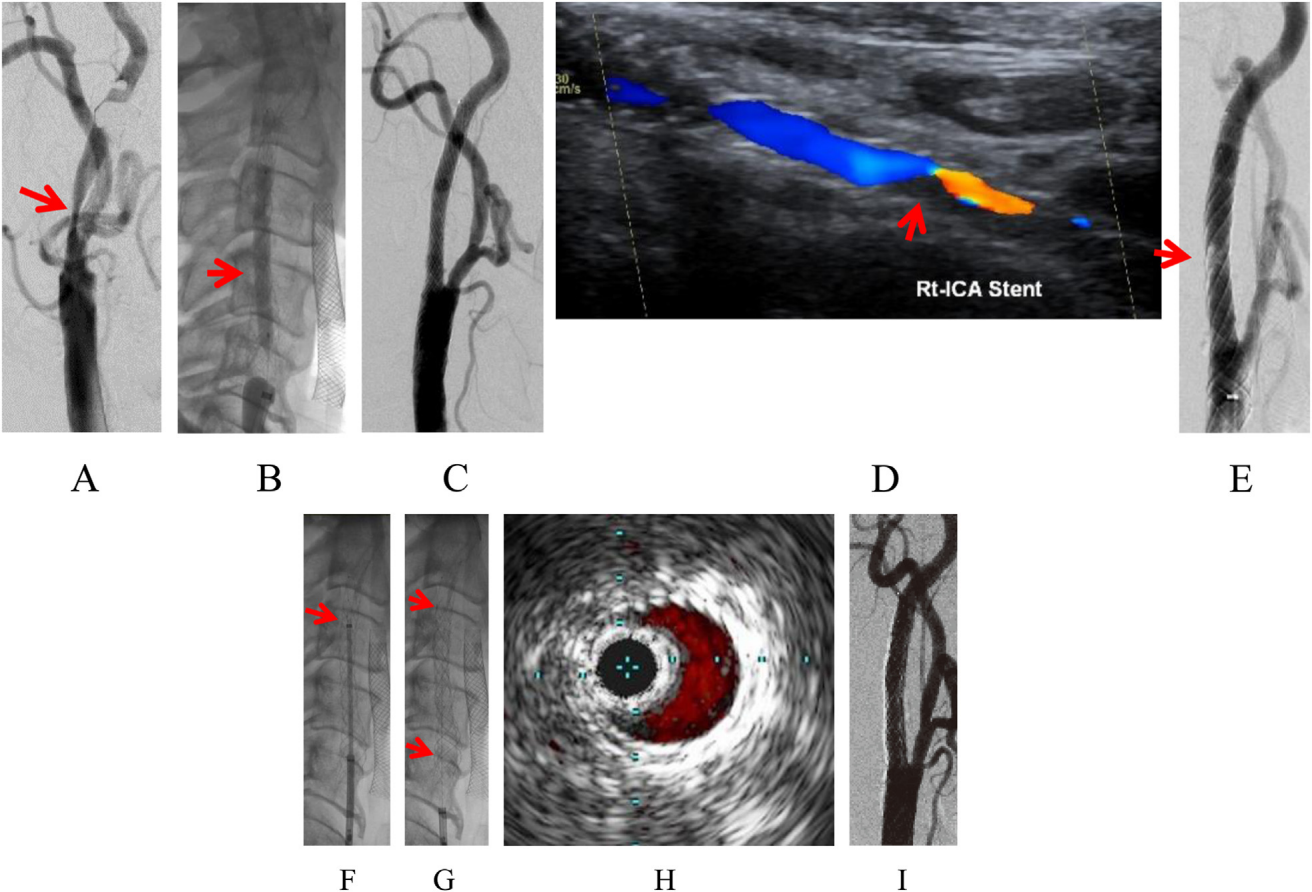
A case of delayed plaque protrusion. (A) Angiogram of the right common carotid artery (frontal view). Severe stenosis is seen at the origin of the right internal carotid artery (arrow). (B) Live image of the right common carotid artery (lateral view). Carotid artery stenting (CAS) using the Casper/Roadsaver stent (CRS) (8 mm × 30 mm) with postdilatation performed using a balloon catheter (4 mm × 40 mm) with OPTIMO and Filterwire EZ to treat internal carotid artery stenosis with unstable plaque. (C) Angiogram of the right common carotid (lateral view). The final angiogram shows good dilatation at the stenotic site, with no obvious plaque protrusion. Intravascular ultrasound at the minimum lumen diameter of the lesion site after stent placement does not show any plaque protrusion. (D) Ultrasound 3 days after CAS shows plaque protrusion (arrow). (E) Angiogram of the right common carotid artery 3 days after CAS (lateral view). Plaque protrusion is evident (arrow). (F) Live image of the right common carotid artery (lateral view). A second stent (CRS 6 mm × 30 mm) is guided inside the first stent because intravascular ultrasound at the minimum lumen diameter of the lesion site 3 days after CAS shows plaque protrusion. (G) Live image of the right common carotid artery (lateral view). The second stent (CRS 6 mm × 30 mm) is positioned in a stent-in-stent manner (arrows). (H) Intravascular ultrasound at the minimum lumen diameter of the lesion site after the second stent placement confirms the absence of plaque protrusion. (I) Angiogram of the right common carotid (lateral view). The final angiogram shows good dilatation at the stenotic site, with no obvious plaque protrusion.
